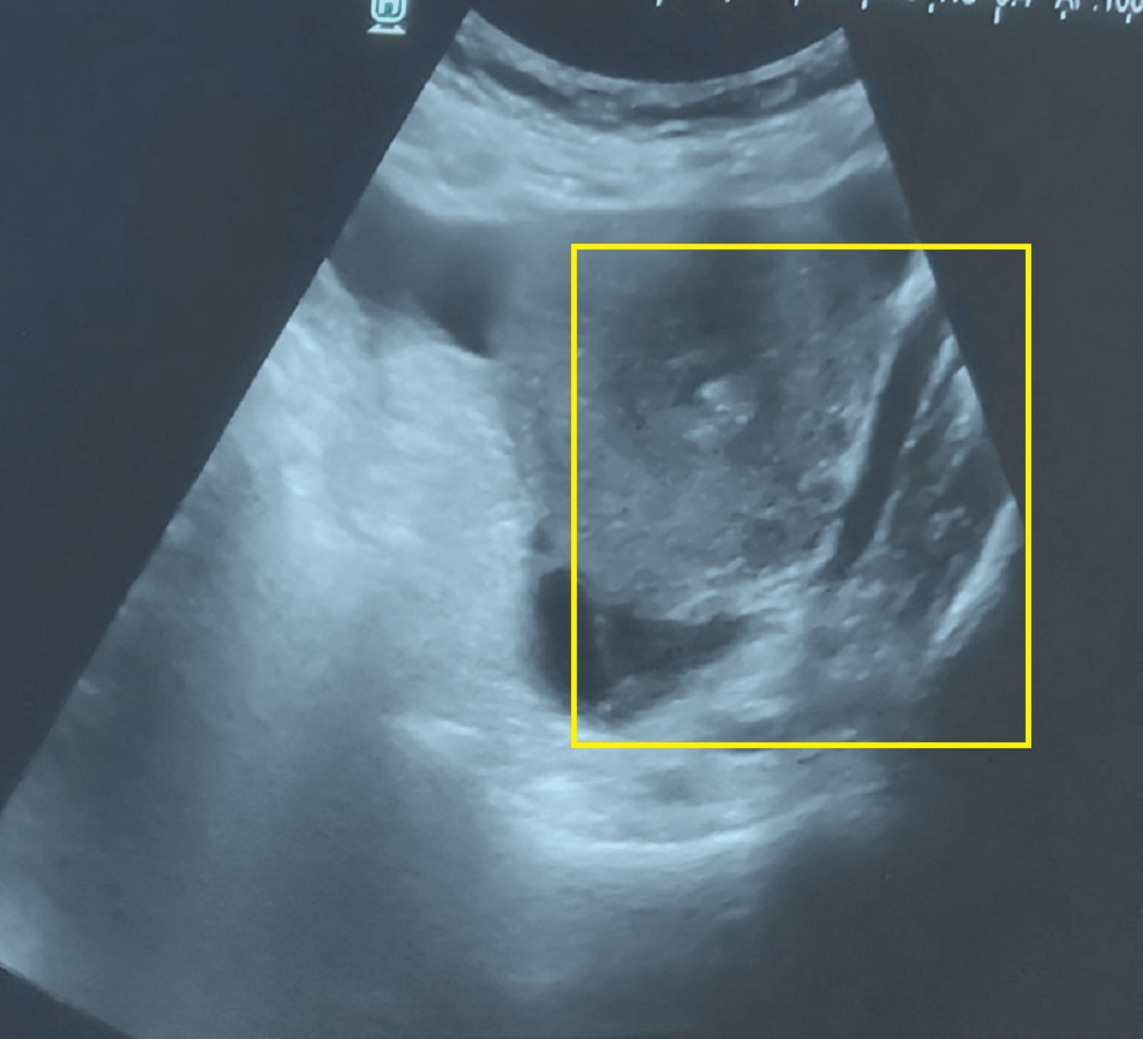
USG image of the patient USG: ultrasound
